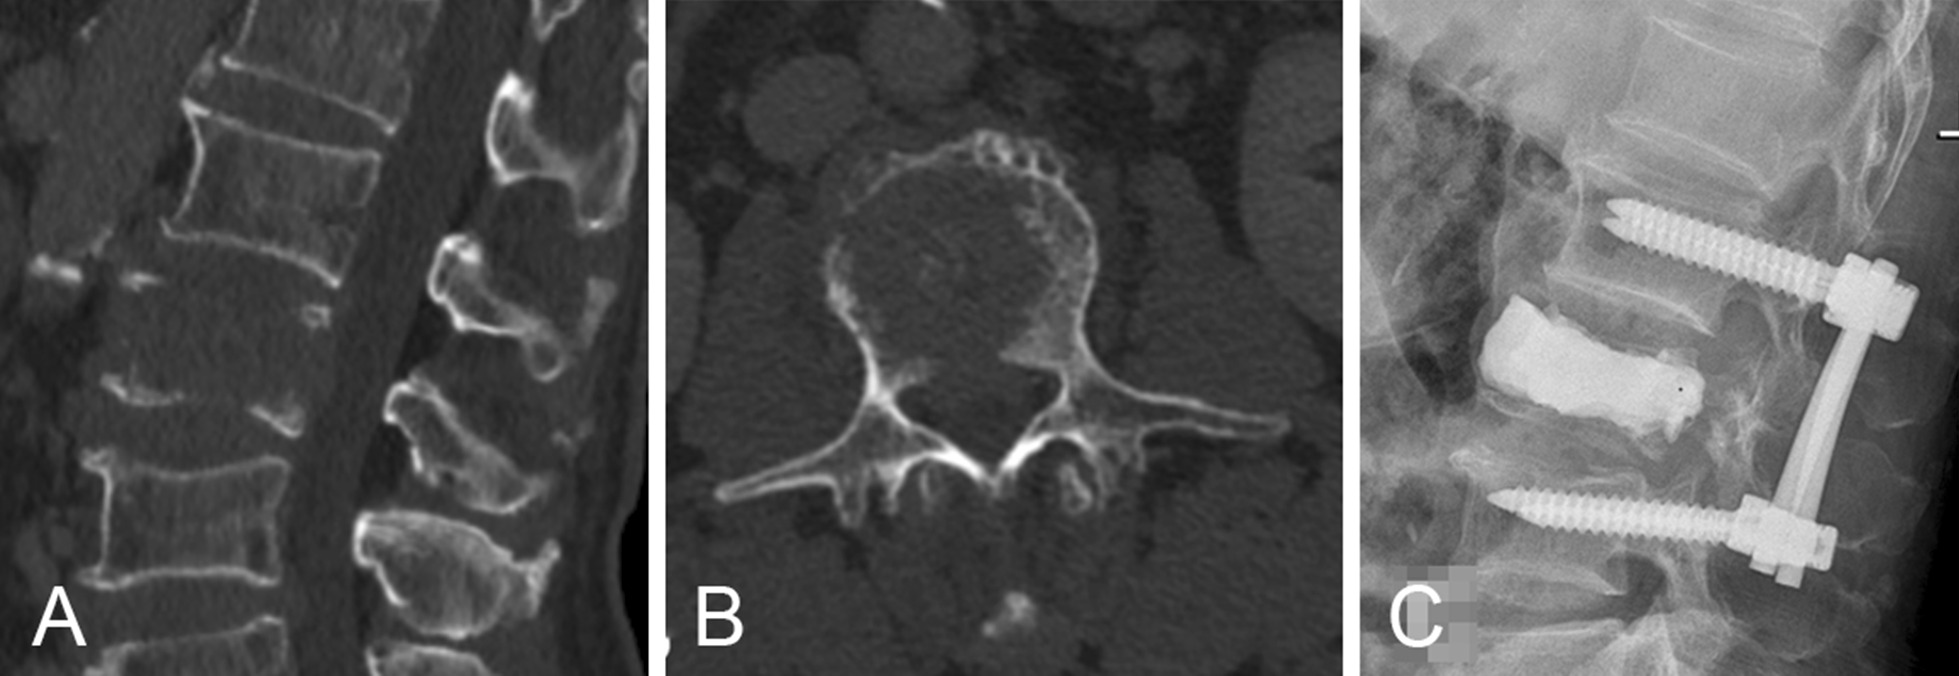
Preoperative CT in an adult patient who had metastatic vertebral fracture with posterior wall damage (A, B); he underwent kyphoplasty combined with pedicle screw fixation (C)
